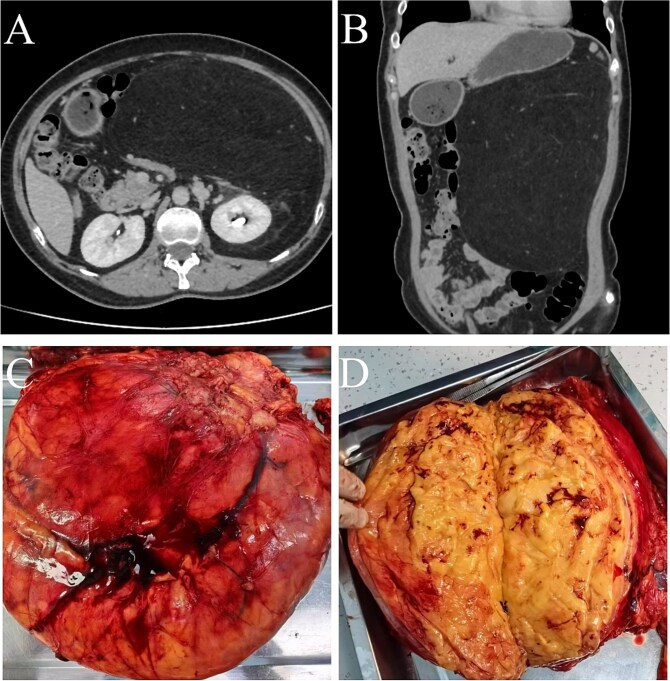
(A and B) CT detected a fatty density lesion in the retroperitoneal space. (C and D) The mass measured 32 × 24 × 7 cm, and had with heterogeneous yellowish mass appearance.
